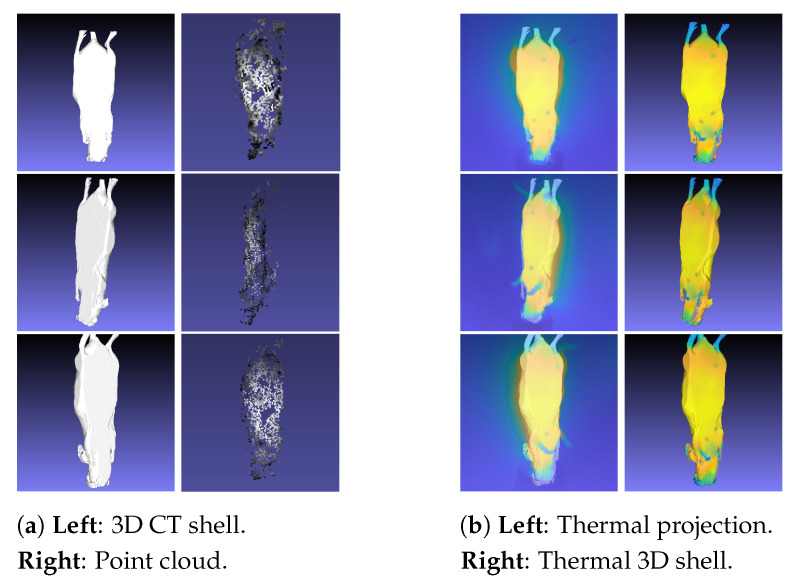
Generation of the thermal 3D shell: (a) Alignment between the 3D CT model and the point cloud. (b) Thermal image projection to generate the thermal 3D shell.
